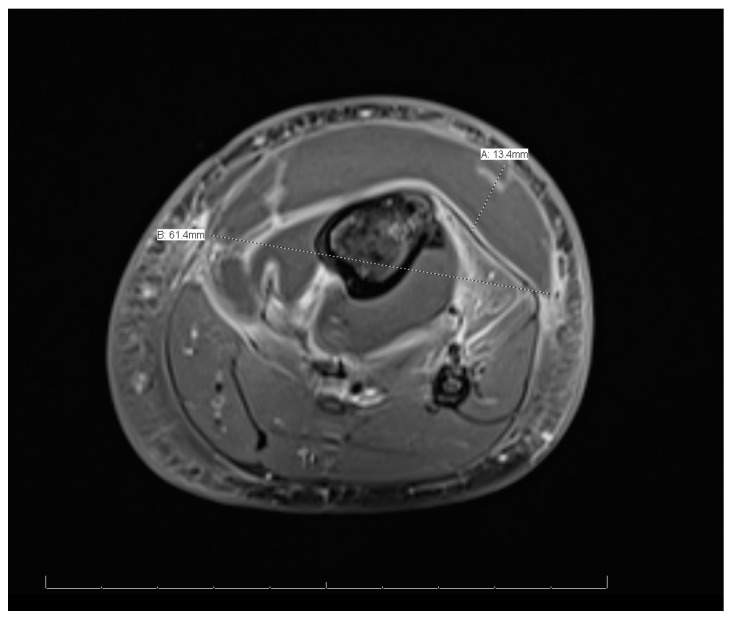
Magnetic resonance imaging of the whole left leg (cross-sectional view).
